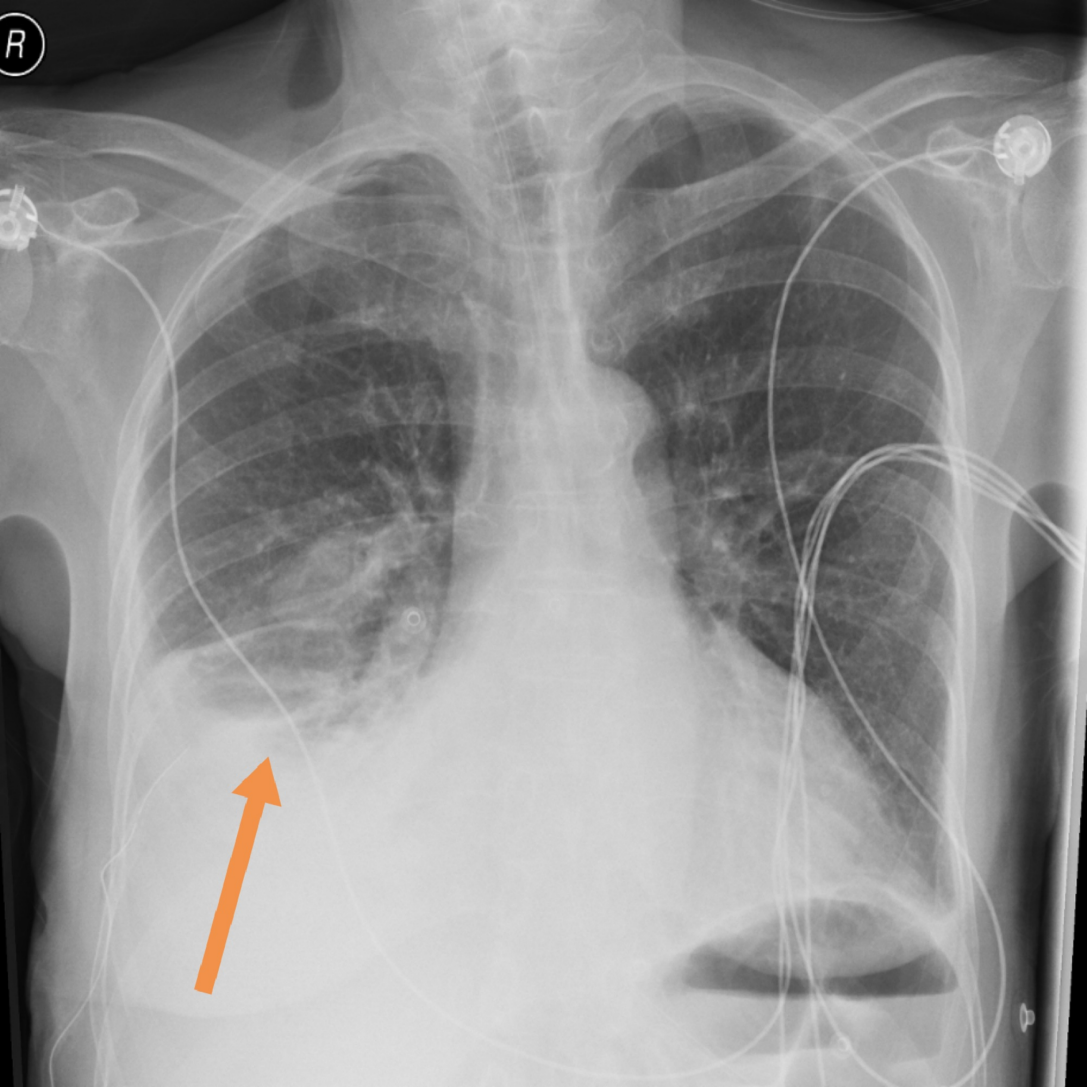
Chest x-ray showing empyema (see arrow)
